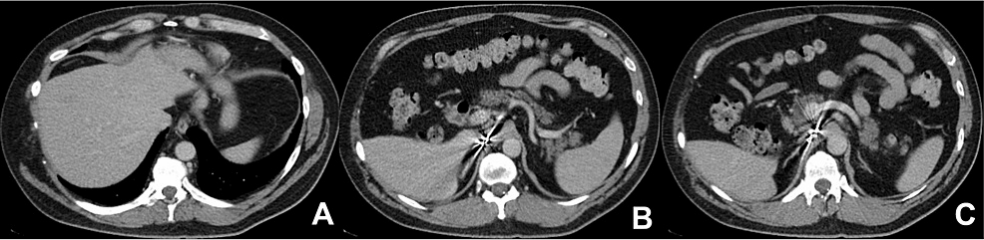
Initial CT of the abdomen and pelvis with contrast without any visible liver or pancreatic lesions
